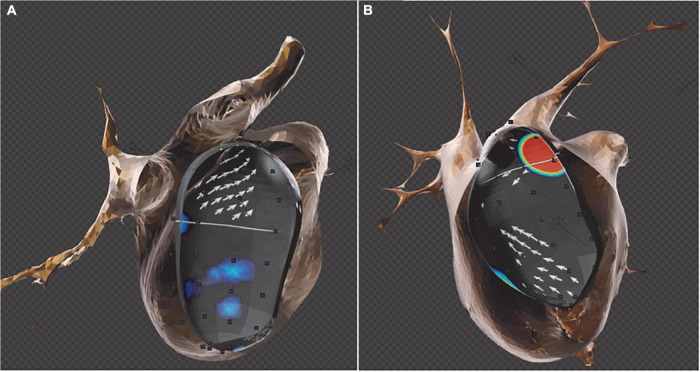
Merged computed tomography (CT) and electrographic flow (EGF) map reconstructions. Sources detected by the EGF software are projected on the CT surface to demonstrate the exact anatomical location within the cardiac chamber. (A) EGF map reconstruction shows electric flow emerging from the left inferior pulmonary vein (LIPV) (see white arrows). (B) EGF reconstructions identify an extra-PV source emerging from the ridge between left atrial appendage–left superior pulmonary vein (LAA-LSPV) (orange patch).
